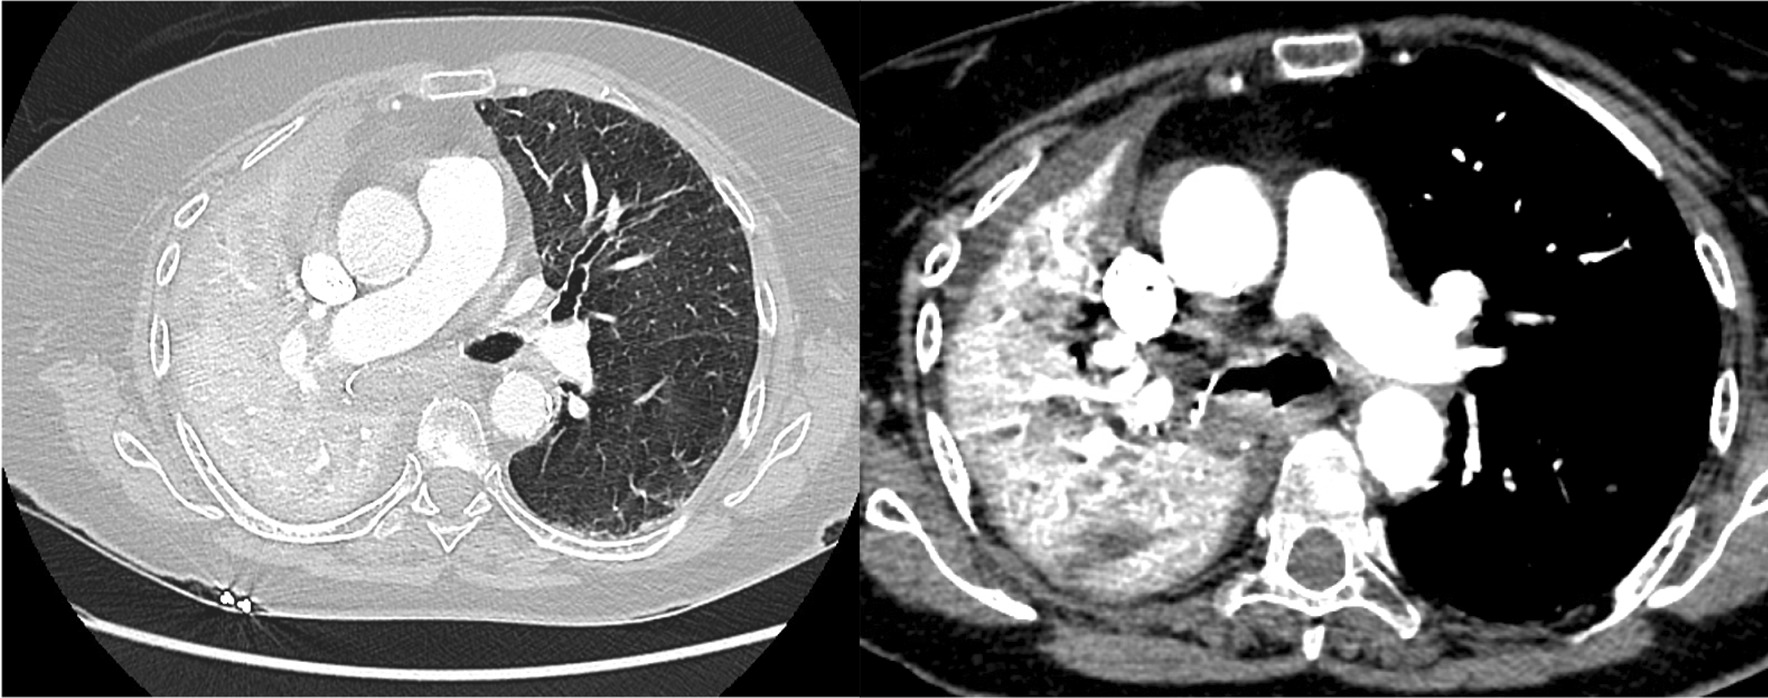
High-resolution computed tomography showing total right lung collapse (case #5)
